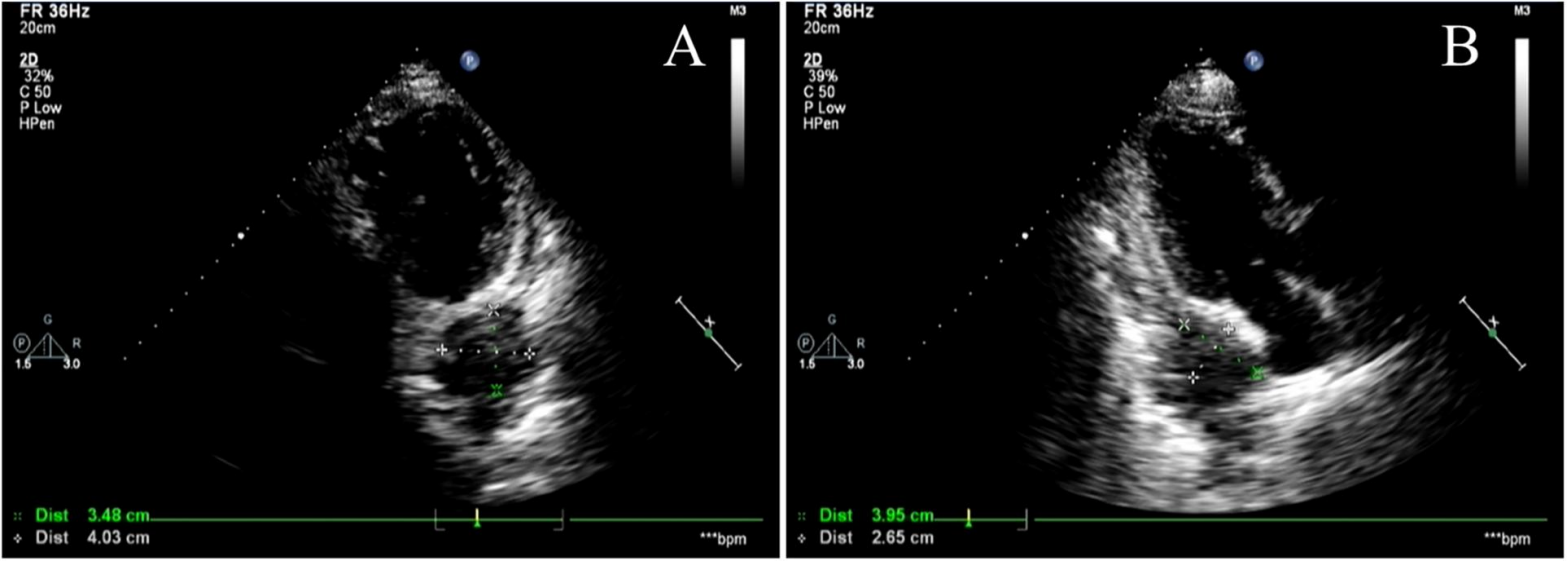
Cardiac Doppler ultrasound. (A,B) show different views of cardiac color Doppler ultrasound revealing a localized hyperechoic lesion measuring approximately 40 × 35 mm in the posterolateral region of the left atrium, exhibiting mild hypoechoic characteristics. Metastatic lesions cannot be excluded.
